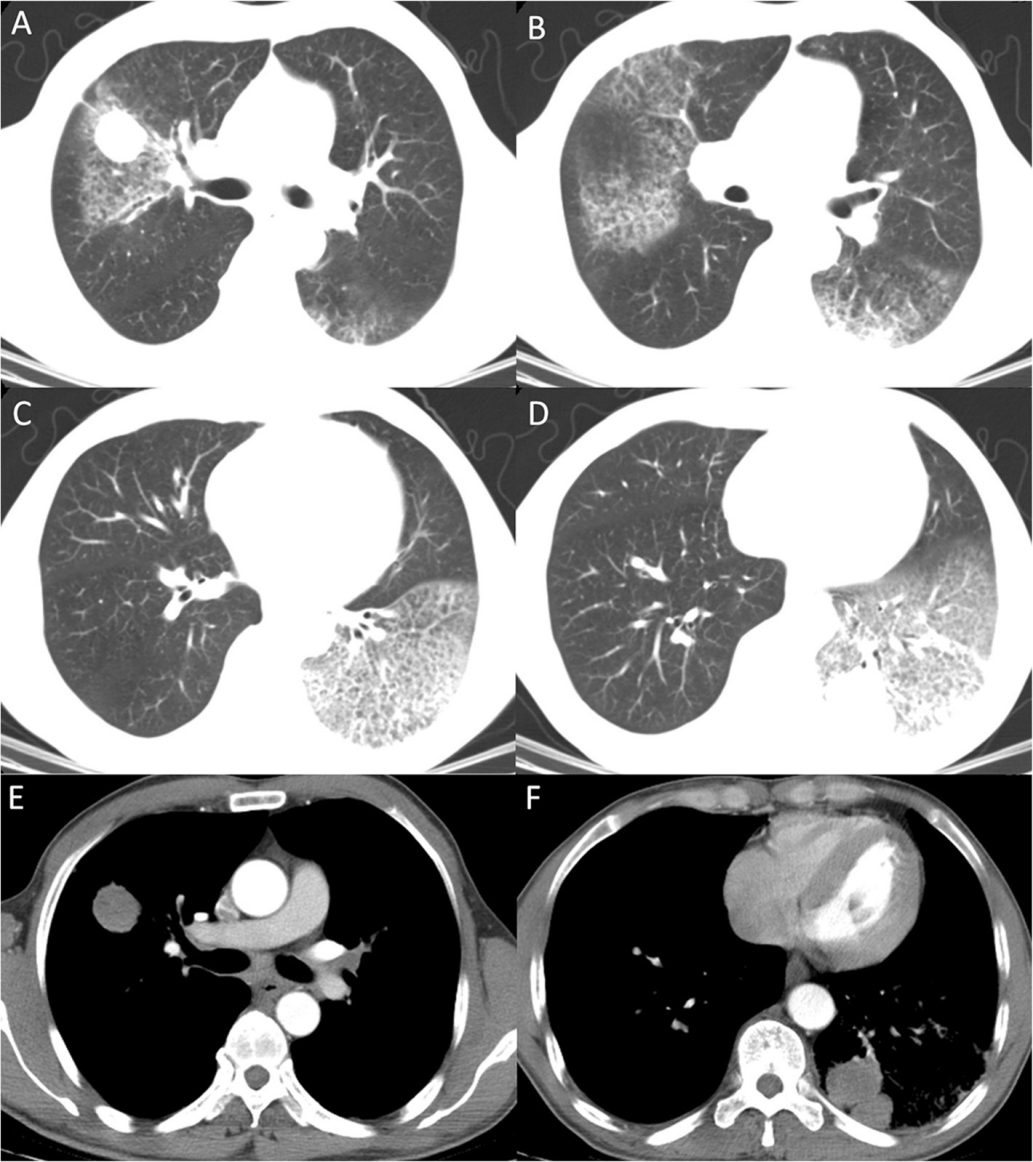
Sixty-year-old man with discrete bilateral nodules with surrounding “paving” pattern. Computed tomography (CT) enhancement scan shows discrete bilateral consolidations with a 28-mm × 24-mm nodule on the right upper lobe (a, e) and a 33-mm × 43-mm subpleural mass with slightly uneven enhancement on the left lower lobe (d, f), accompanied with intralobular interstitial thickenings shown as a surrounding bilateral paving pattern (b, c)
